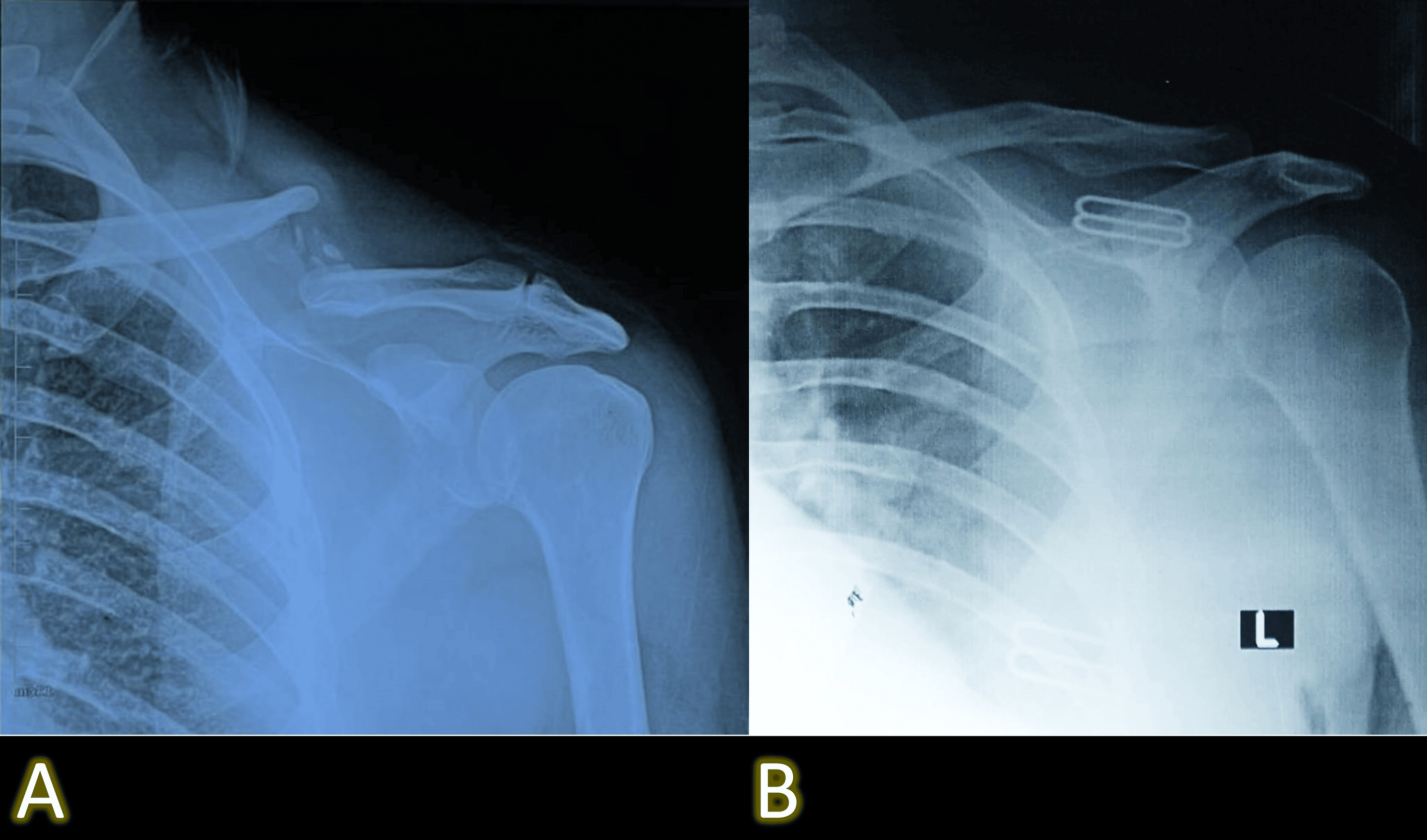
Radiographic comparison demonstrating nonunion and eventual healing following conservative management. (A) Nonunion evident at 24-week follow-up. (B) Healed fracture at 52-week follow-up.
